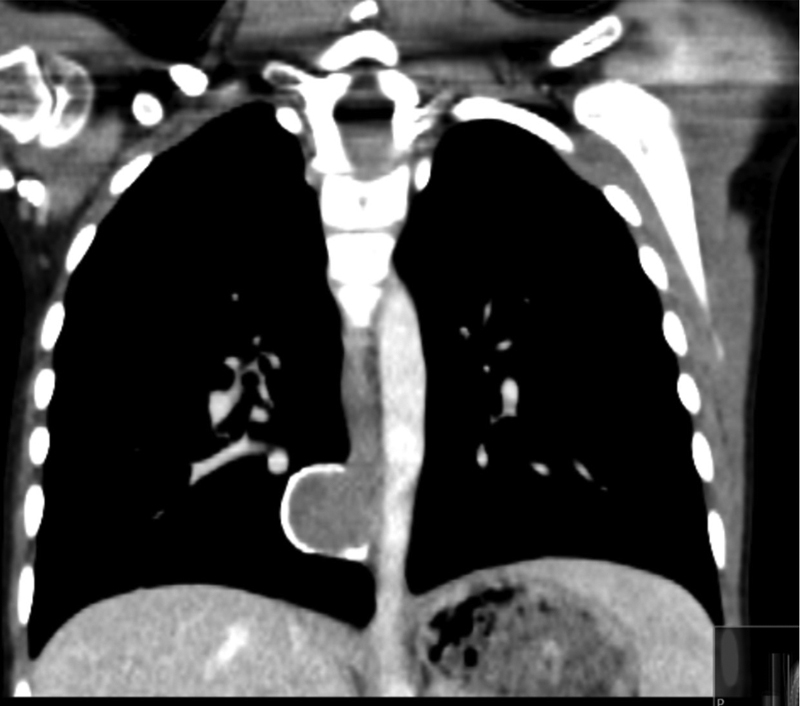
Computed tomography scan.
